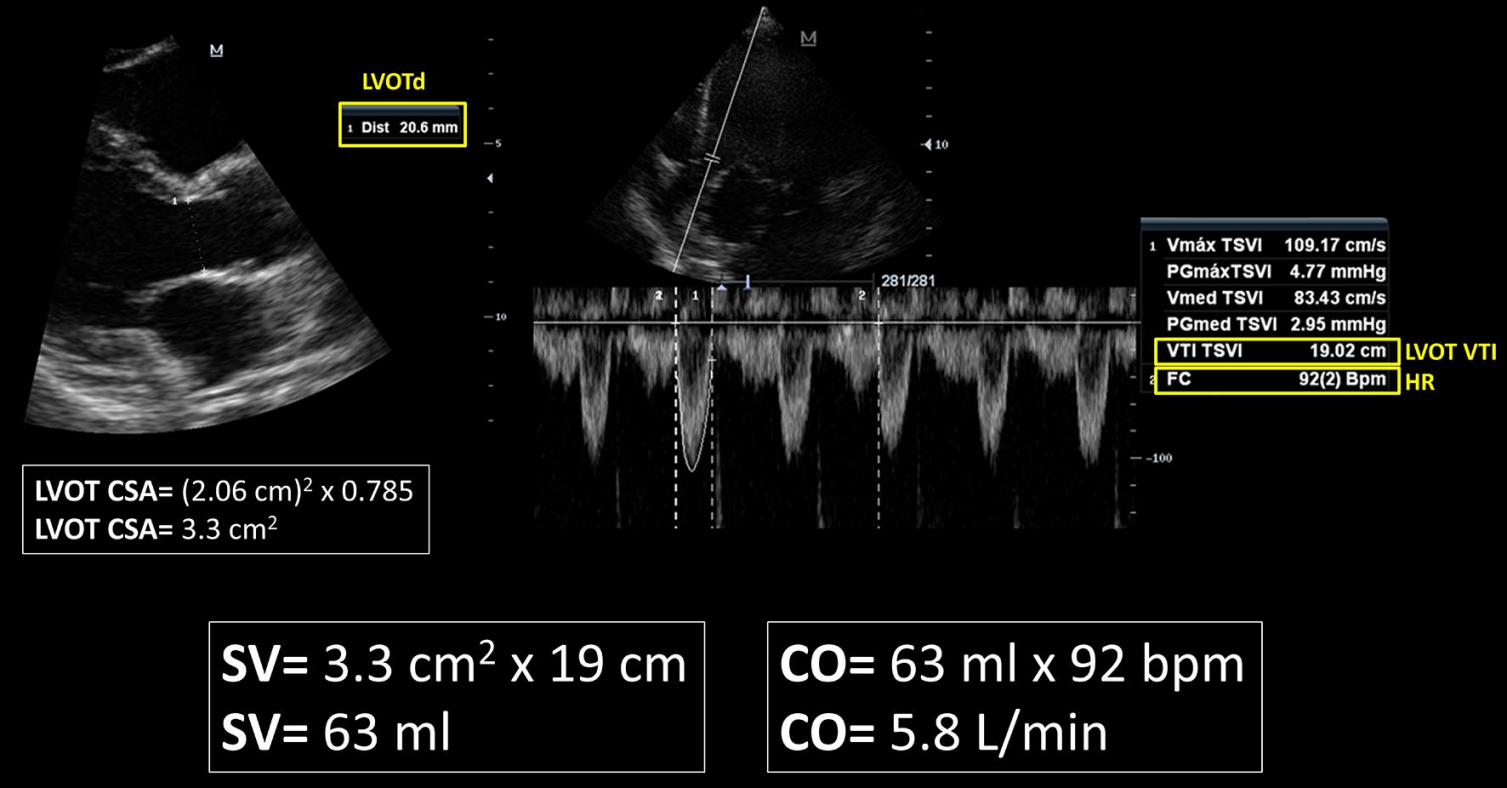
Calculation of the stroke volume (SV) and cardiac output (CO) by transthoracic echocardiography in a critically ill patient without hemodynamic compromise. LVOT: left ventricular outflow tract; d: diameter; CSA: cross-sectional area; VTI: velocity–time integral; HR: heart rate
